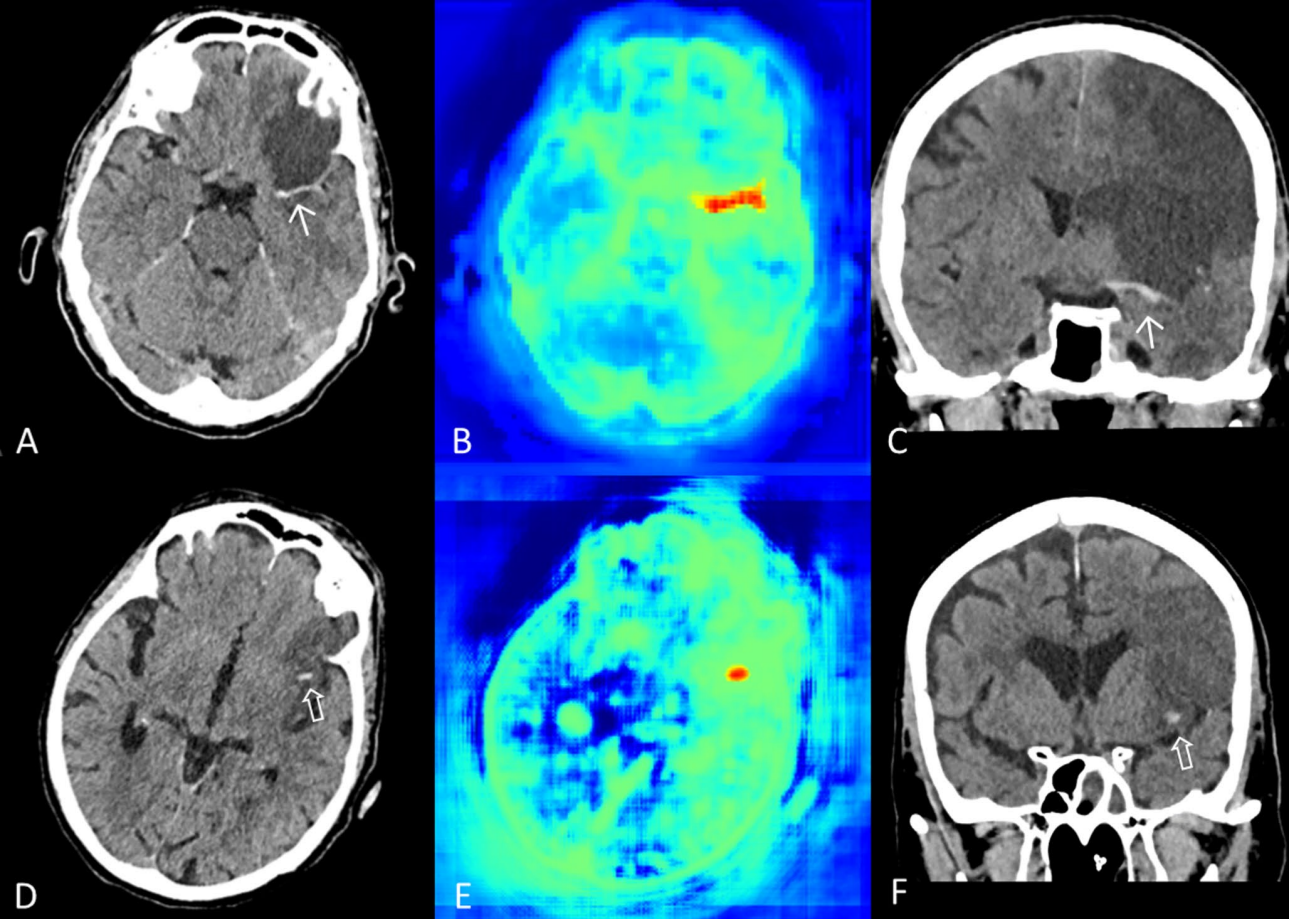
The top row shows a patient after a futile mechanical thrombectomy, with a hyperdense vessel due to a remaining long-segment occlusion of the left carotid-T and middle cerebral artery (see thin white arrows in axial [A] and coronal [C] non-contrast enhanced CT images), which was falsely identified as hemorrhage by the AI algorithm (B), but correctly acknowledged in the radiological report. The bottom row presents a patient after mechanical thrombectomy with a small basal intracerebral hemorrhage (see hollow white arrow in axial [D] and coronal [F] non-contrast enhanced CT images), which was correctly marked by the AI algorithm (E). The radiological report for the patient in the bottom row did not acknowledge the presence of this hemorrhage. Note the similarity in location and configuration of these hyperdense structures in both cases
